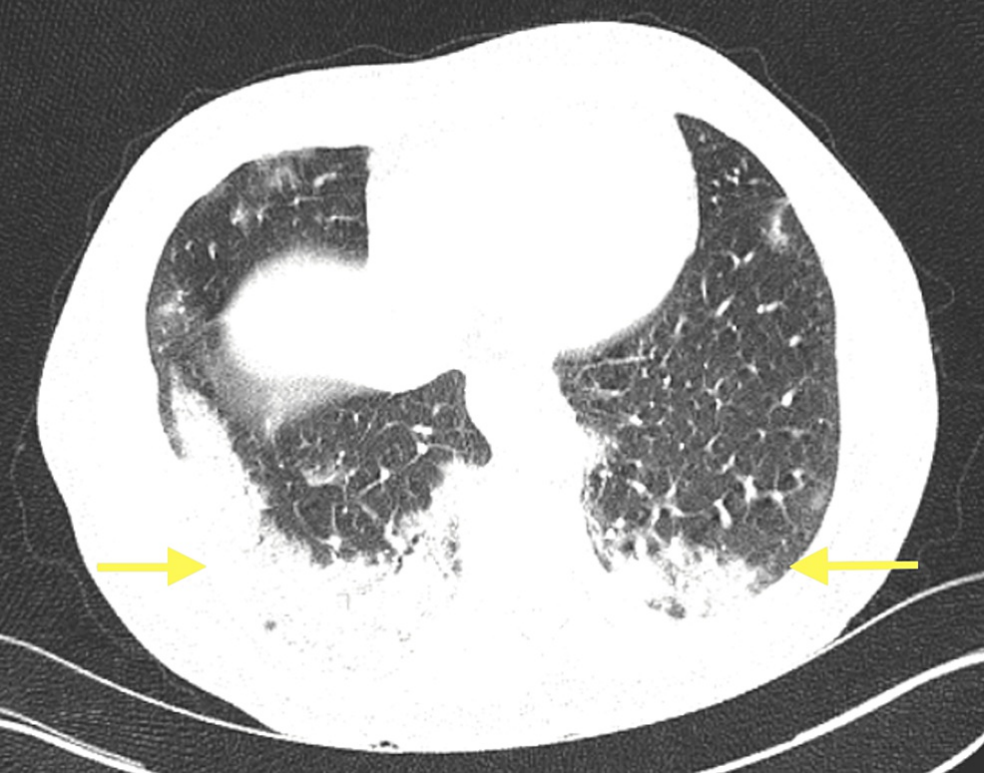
Pneumonic infiltrates in the bilateral basal zone in thorax CT
